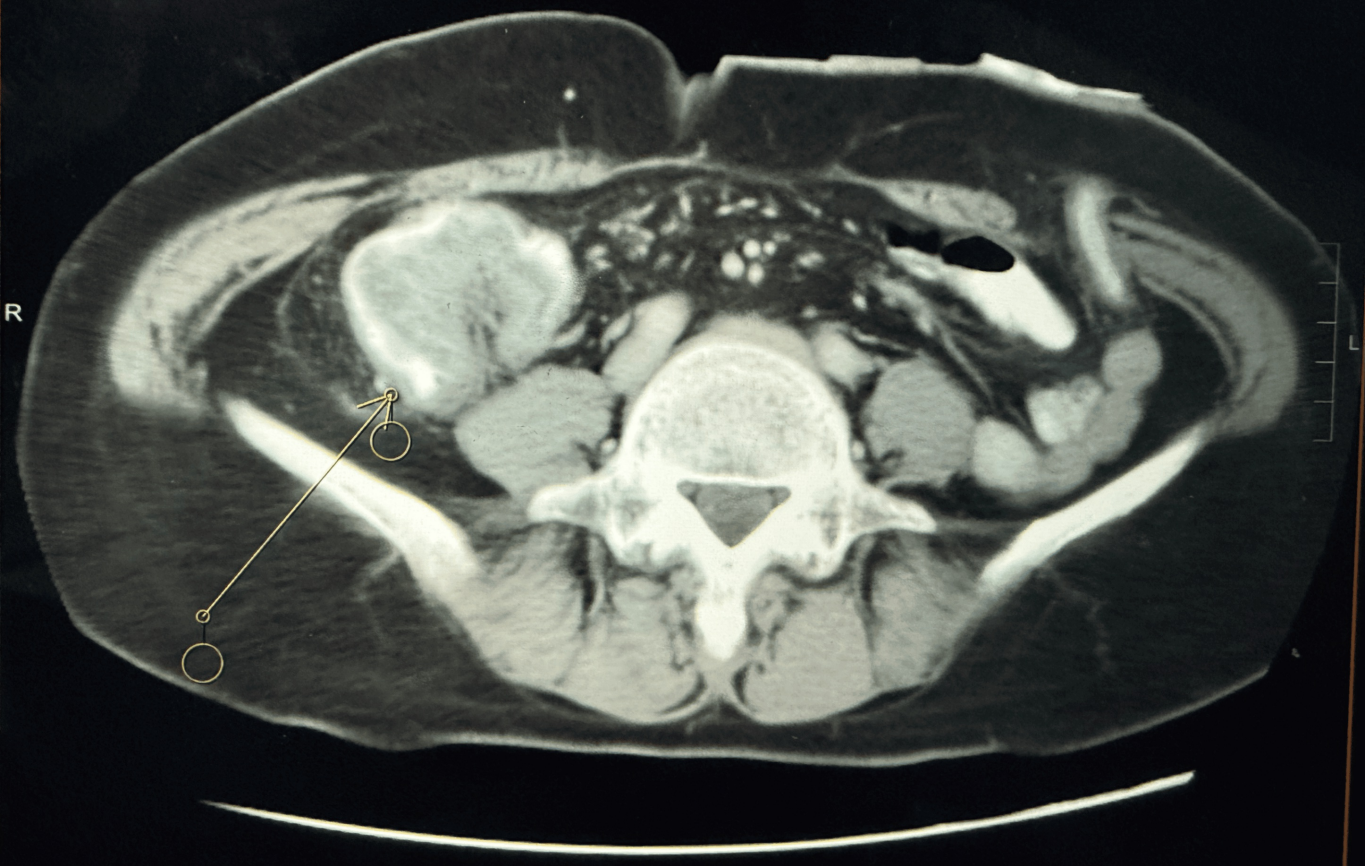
Computed tomography showing 4.5 cm right side CA colon. The arrow points to the recurrent mass. CA: adenocarcinoma
